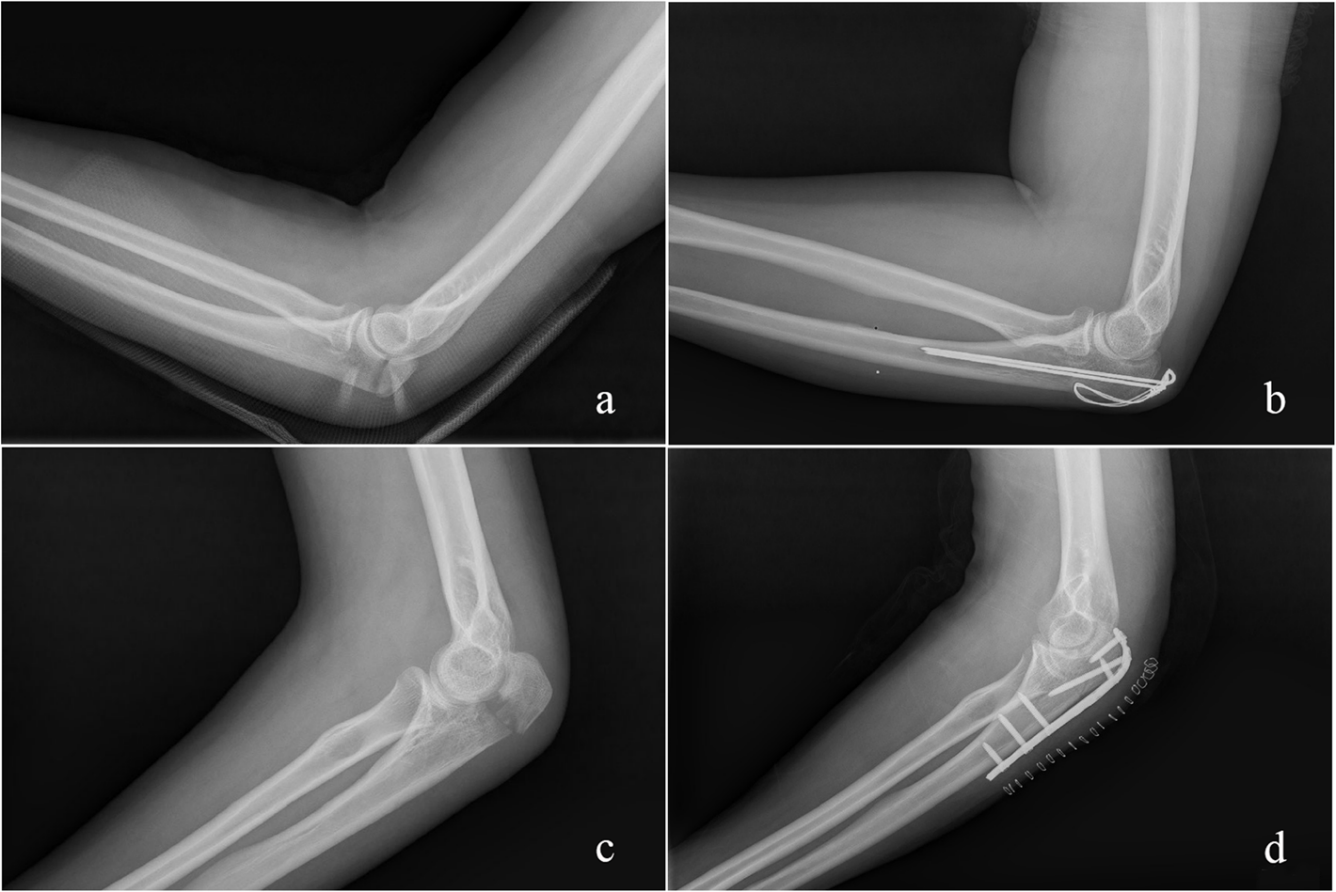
A Preoperative lateral radiograph of elbow demonstrating Mayo 2A olecranon fracture. B Postoperative radiograph after tension band wiring. C Prepoperative lateral radiograph of elbow demonstrating another Mayo 2A olecranon fracture. D Postoperative radiograph after plate fixation. This figure comes from the corespondent author’s research team
